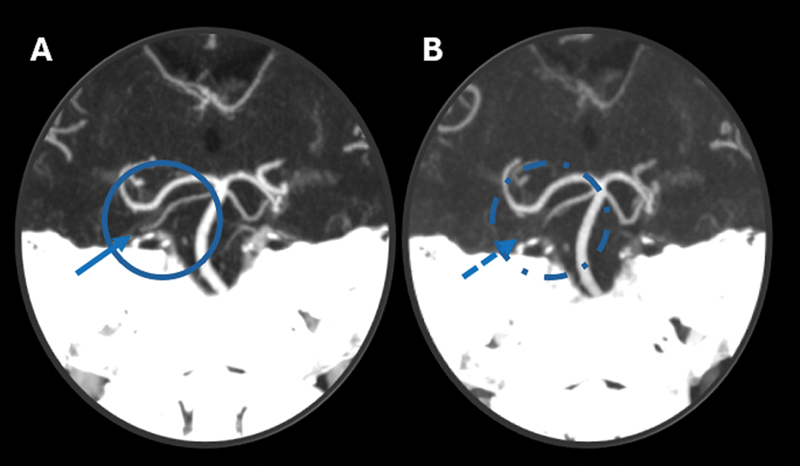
Computed tomography angiography (CTA) scan of the head, coronal maximum intensity projection (MIP) reconstruction with zoom-in of the vertebrobasilar system. (
